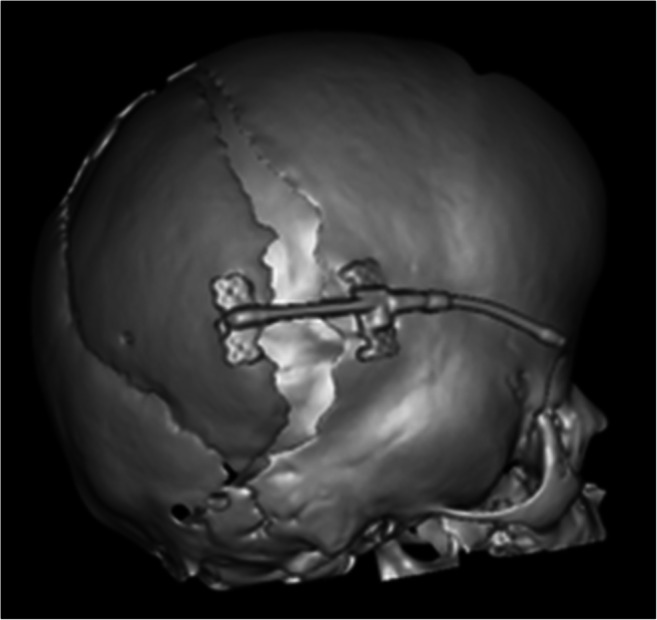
A 3D CT image showing the so-called gull wing complication, where the bone flap bends at the patent lambdoid sutures during the distraction
